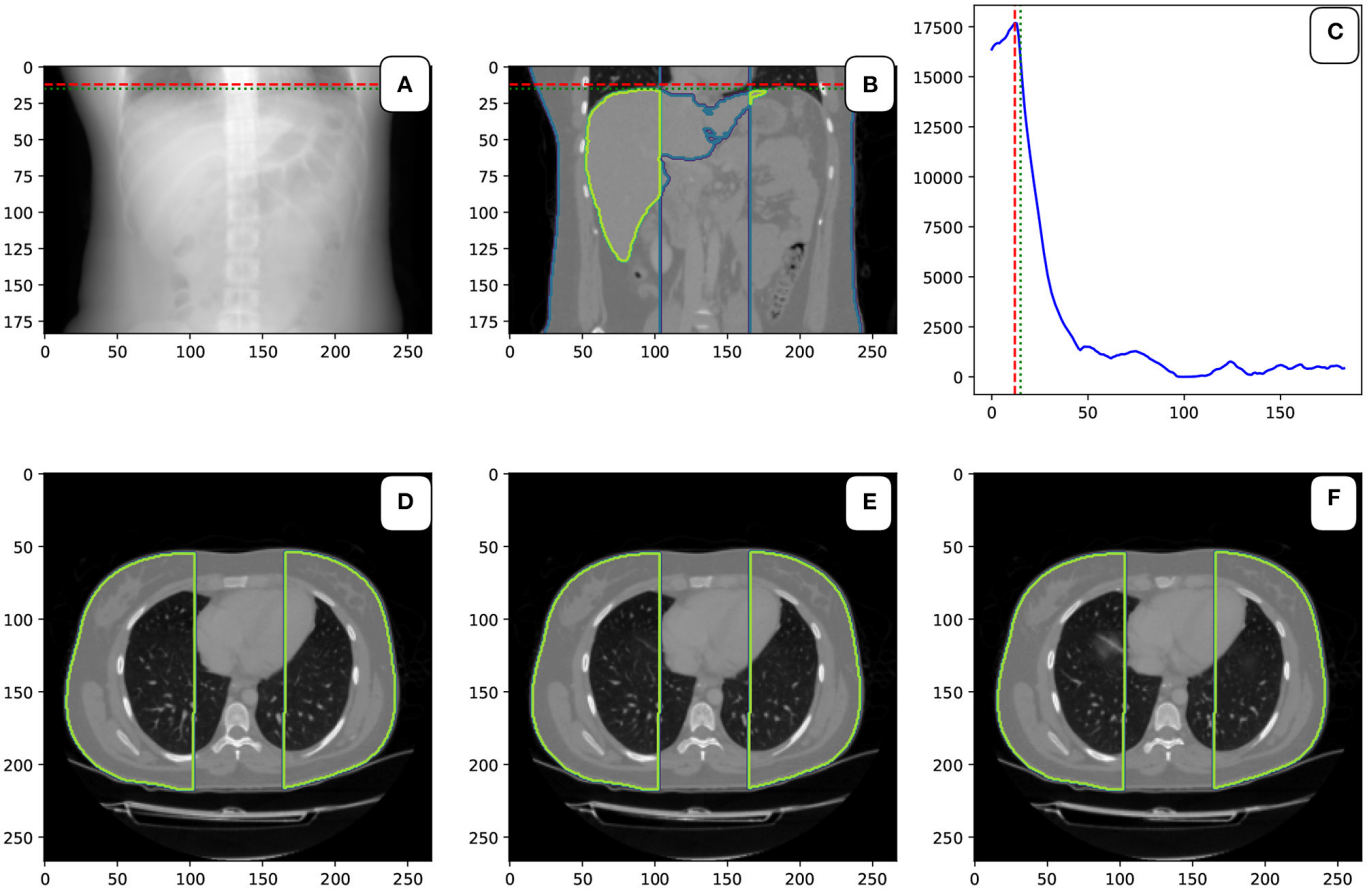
Localization of the top of the diaphragm: Image (A,B) in the first row show the CT scan's coronal view. The green and blue delineation show the liver and the mask. The red dashed line is the detected level of the top of the diaphragm. The green dashed line shows the top level of the liver. The image (C) in the first row is the plot with the area of the masked cavity in square mm. Images (D–F) show three consecutive axial slides of the CT scan. In the middle (E) is the slice from the top level of the diaphragm can. On the left (D) is the slice above the diaphragm. The right image (F) shows the slide under the top level of the diaphragm. A small “shadow” of the diaphragm can be seen in this image.
